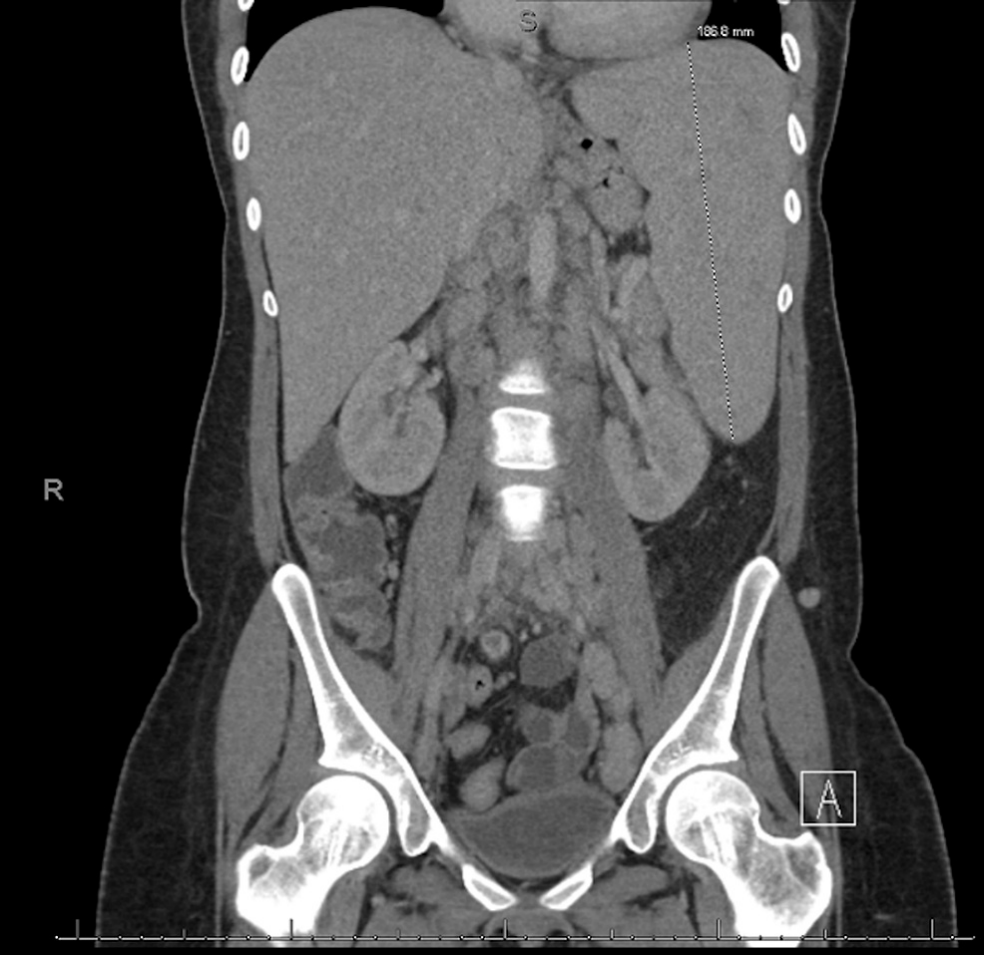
CT abdomen and pelvis with IV contrast showing splenomegaly (186.8 mm)
